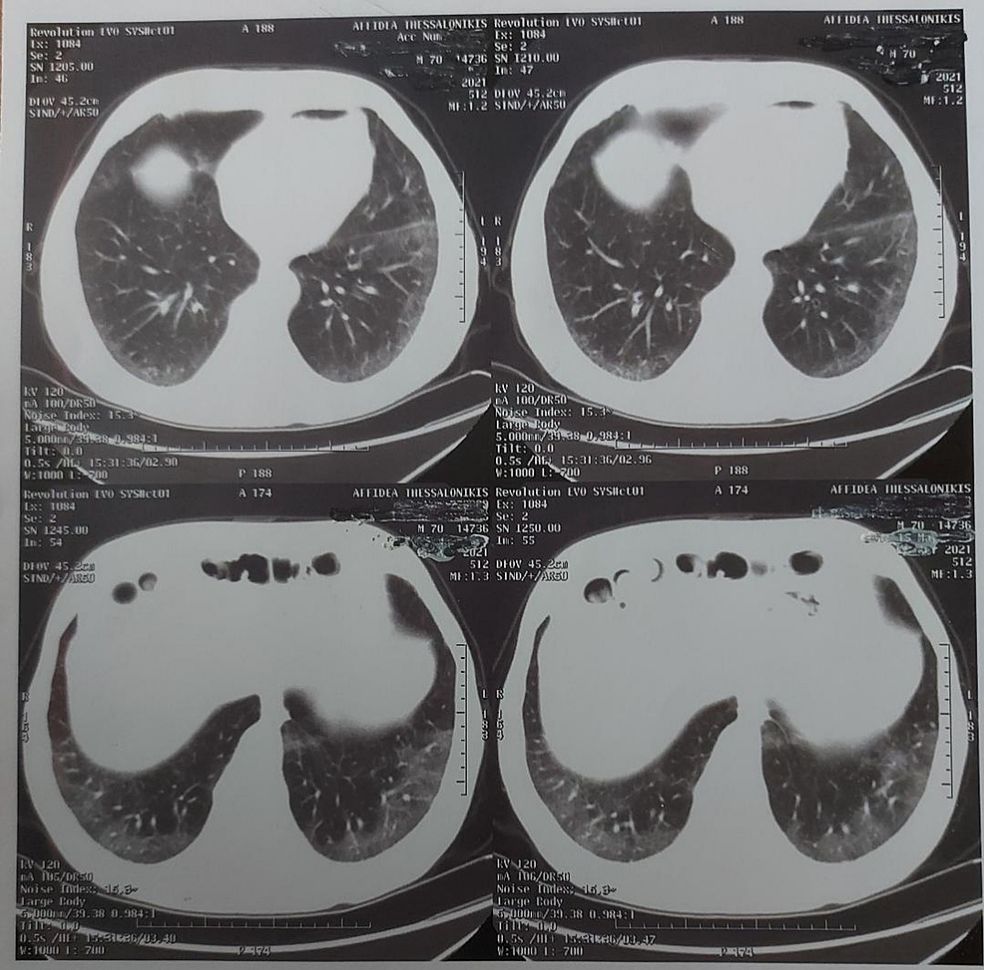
The CT scan of the chest demonstrating multiple areas of ground-glass opacities located predominantly peripherally and posteriorly
